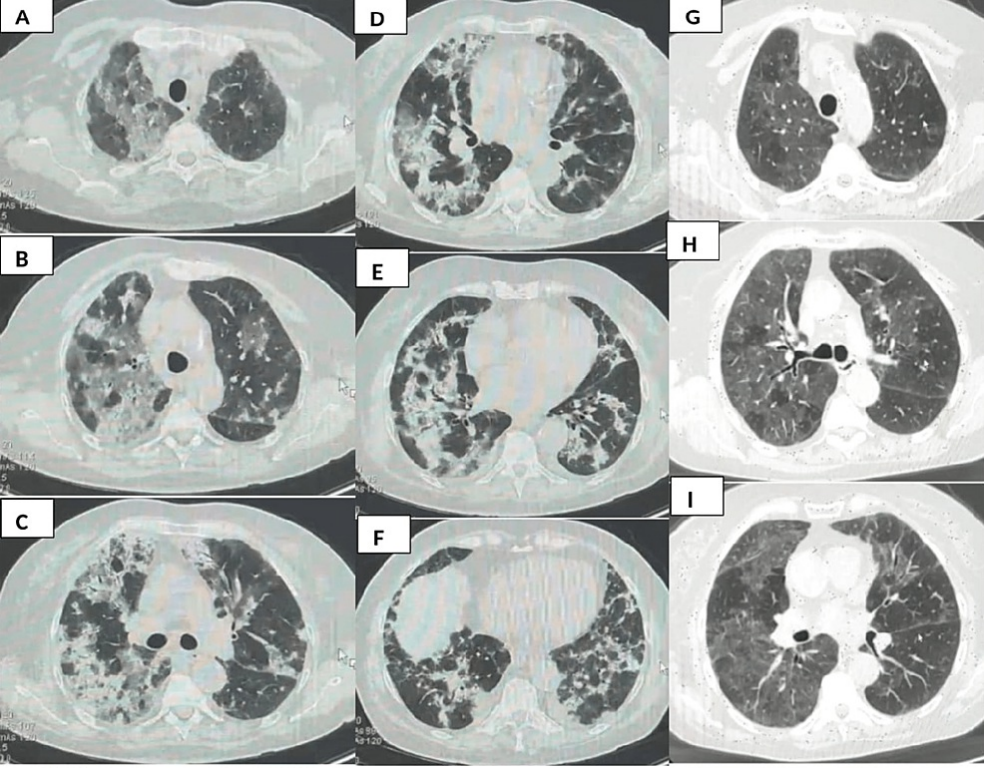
A: Time zero chest CT scan showing areas of bilateral apical centrilobular consolidation with thickening of the pulmonary reticular interstitium of the intra- and interlobular septa, with predominance in the right apical areas. B: Time zero chest CT scan showing areas of bilateral apical centrilobular consolidation with thickening of the pulmonary reticular interstitium of the intra- and interlobular septa, with predominance at the right apical zones with presence in both lungs at the submantellar zones of solid centrilobular consolidations. C: Chest CT scan at time zero showing areas of right perilobular consolidation with thickening of the reticular pulmonary interstitium of the intra- and interlobular septa and prevalence in the right middle field of areas of solid centrilobular consolidation. D-E-F: Time-zero chest CT scan showing areas of peri- and mid-basal centrilobular consolidation prevailing on the right, thickening of the reticular pulmonary interstitium intra- and interlobular septa, and predominance in the mid-right field of areas of solid centrilobular consolidation. G-H-I: Chest CT scan carried out at three months of therapy with an oral corticosteroid, inhaled corticosteroid, and a long-acting bronchodilator, showing apical, middle, and basal areas almost complete regression of the areas of consolidation. CT: computed tomography
